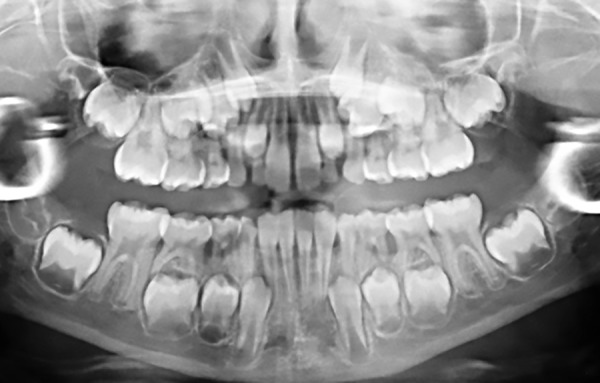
Radiographic situation before therapy of the OS (Orthopantomogram)
